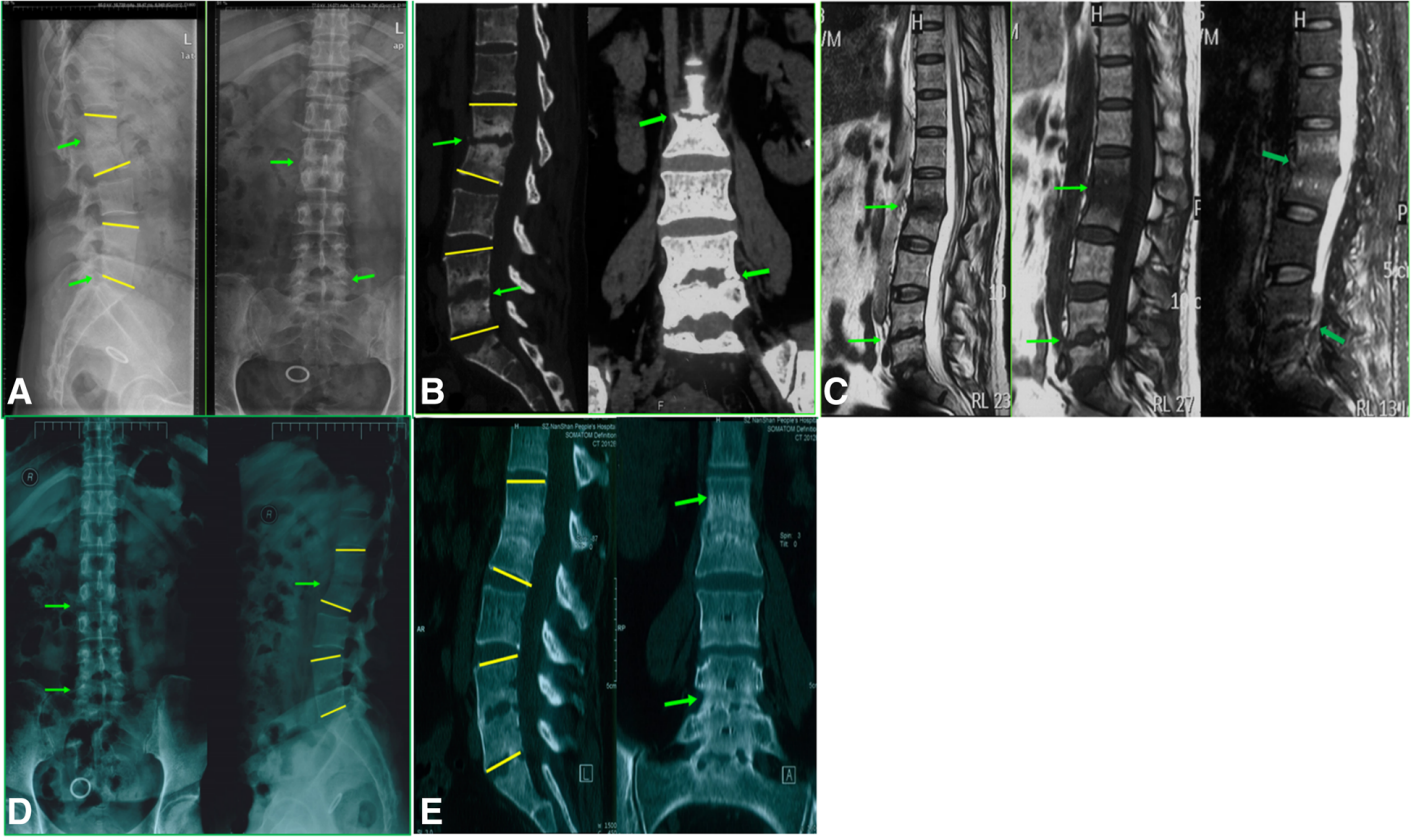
These are radiology data for a 44-year-old female with mild spinal tuberculosis at L1/L2 and L4/L5. The patient was treated with standard first-line antituberculosis drugs for 18 months. a The lateral view of X-ray shows that, before the treatment, there was bone destruction between the L1/L2 and L4/L5 vertebral (*arrow*) and the Cobb angles (*yellow lines*) for the local kyphosis were 20.3° and 10.5°, respectively. The anterior and posterior views show significant intervertebral space reduction (*arrow*) of L1/L2 and L4/L5. b The CT images before the treatment show bone destruction and intervertebral space reduction (*arrows*) at L1/L2 and L4/L5 levels, and the Cobb angles (*yellow lines*) of kyphosis were 22.6° and 8.5°, respectively. c The MRI images before the treatment show significant edema and absence of normal intervertebral signal at L1/L2 and L4/L5 level (*arrows*), but there is no compression on the spinal cord or nerve roots. d The anterior posterior and lateral views of X-ray show that, after 18 months of standard chemotherapy of first-line drugs, there is no progression of bone destruction or vertebral collapse, and there is formation of bonny bridging (*arrows*) between the L1 and L2 vertebra, which indicated possible simultaneous spinal fusion. The Cobb angles of local kyphosis (*yellow lines*) were 20.8° and 11.3°, respectively. e Two years after the 18-month standard chemotherapy treatment, the CT images show solid fusion at the L1/L2 and l4/L5 levels, and the Cobb angles of local kyphosis (*yellow lines*) were 19.8°and 9.8°, respectively. There was no significant progression of local kyphosis compared to those of pretreatment
